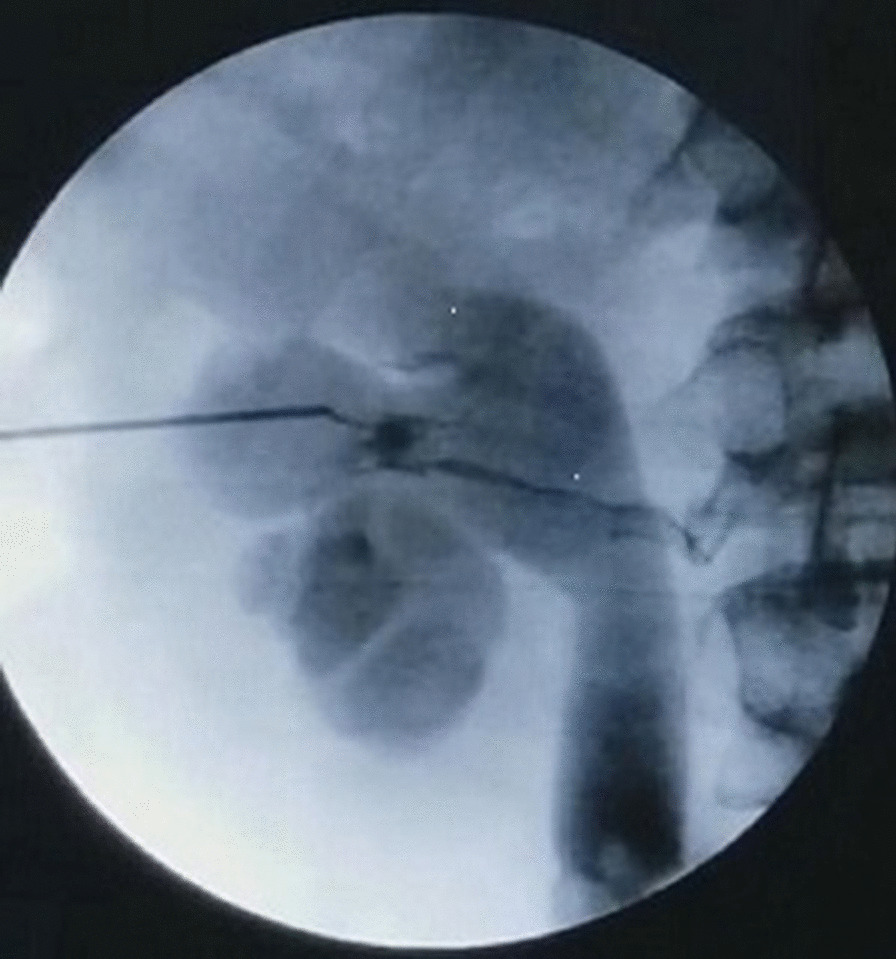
The radiograph of the kidneys, ureters and bladder revealing the left renal vein, the collecting system, and the suboptimal position of the puncture needle tip
